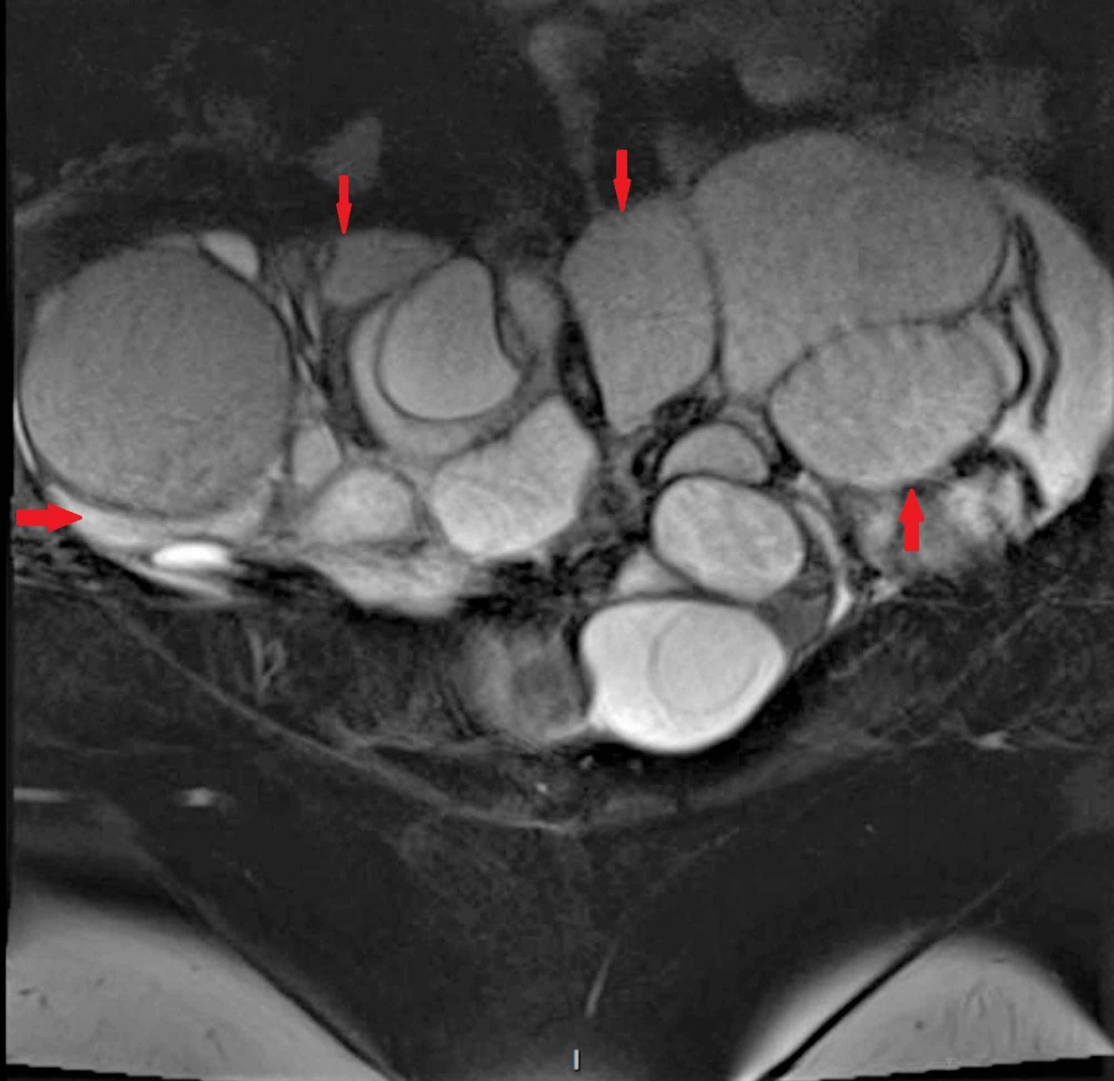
Coronal section of the patient's pelvic MRI showing severely enlarged bilateral ovarian masses with numerous cystic spaces separated by thin septations
